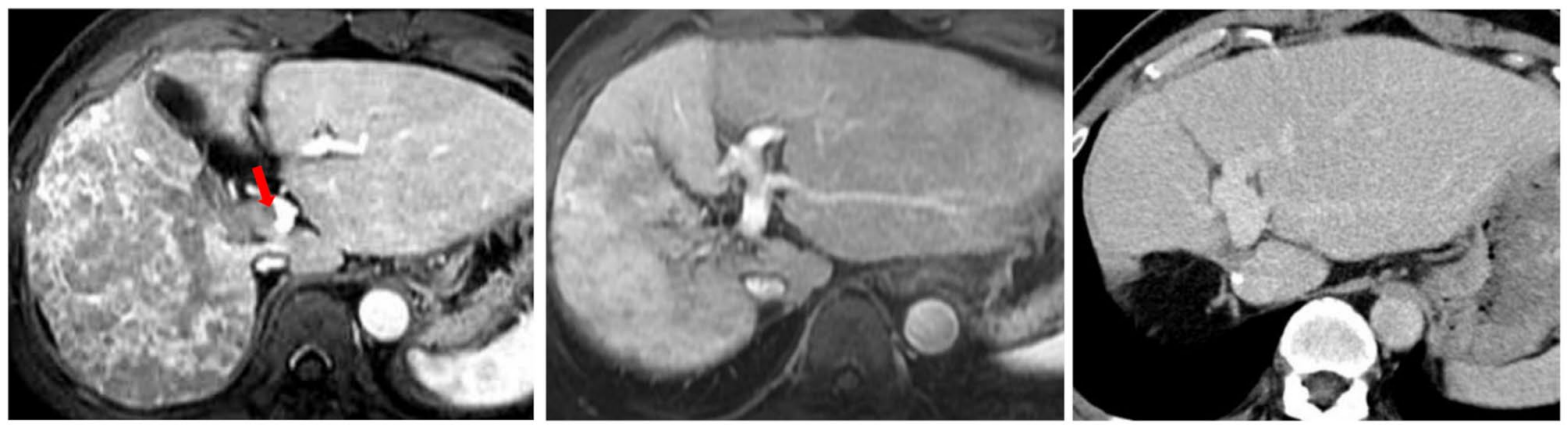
A 56 year-old male patient with a confluent 14 cm HCC in the right lobe liver. A: Red arrow indicates tumor invasion into the main portal vein (Vp4). B: After 3 courses of HAIC neoadjuvant therapy with PR, this patient underwent right hepatectomy. C: 5-year follow-up CT image showed no evidence of intrahepatic recurrence
